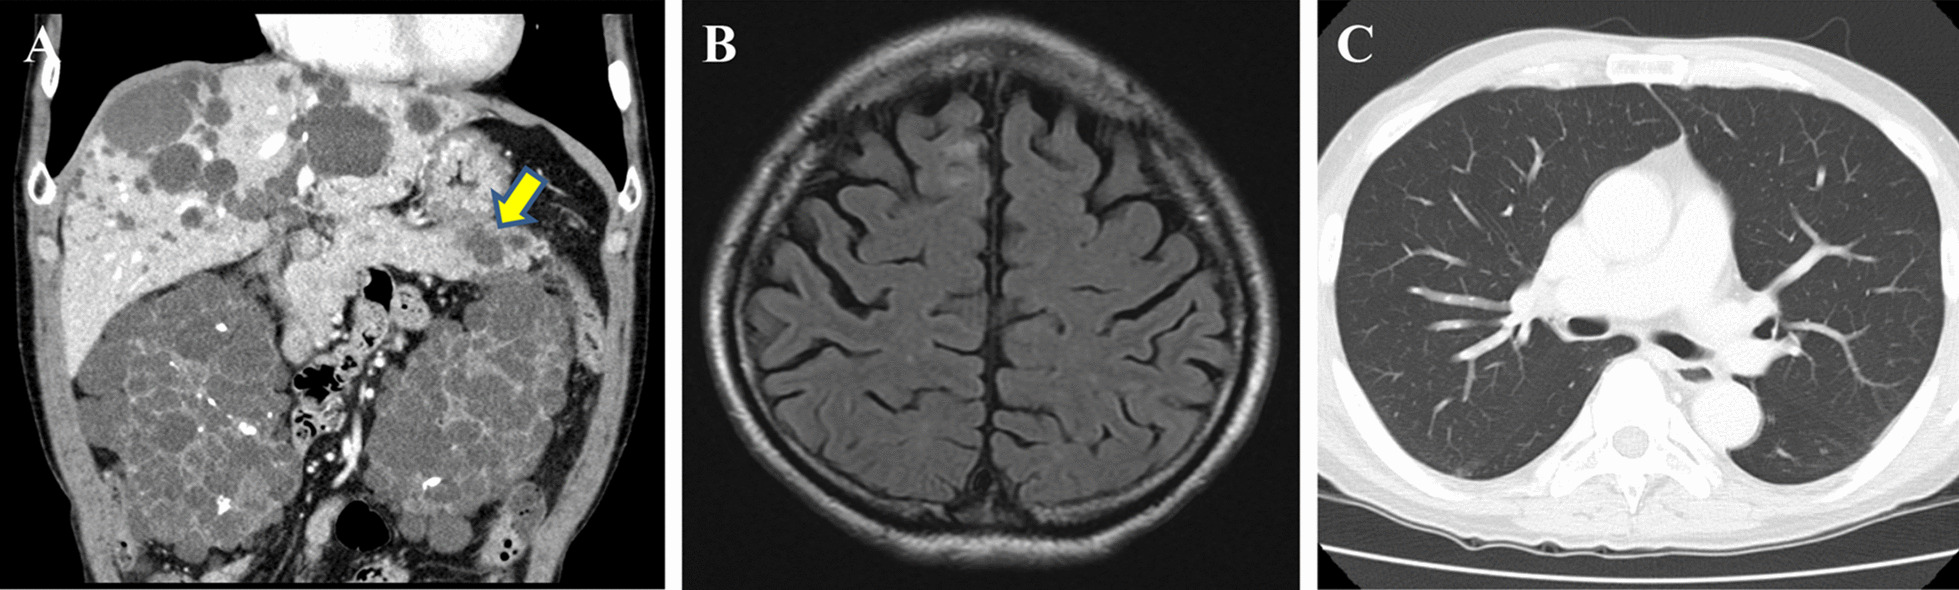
Radiological images at the time of diagnosis. a Computed tomography revealed a low-density mass in the tail of the pancreas (yellow arrow), and polycystic liver and kidney. b No metastases were observed in the lungs. c MRI image revealed no metastasis in the brain
